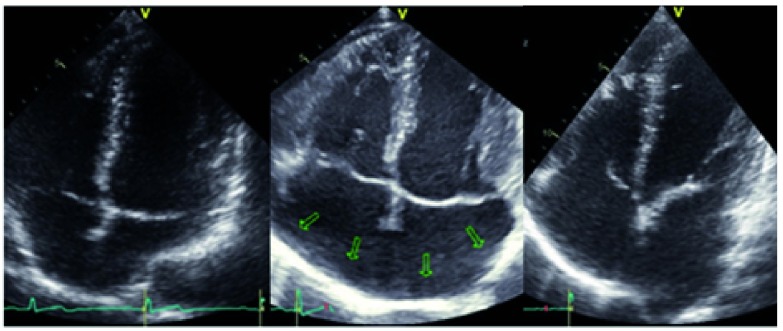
Pathological cardiac remodelling (especially of right and left atrium) of a 48 years old triathlete with EIAH. In 2011, the participant showed a normal size of the right and left atrium. An atrial enlaragement occured 2014 (arrows) after a period of high intensity training (echocardiography in 4 chamber view during atrial fibrillation). Even after cardioversion (2014) into sinus rhythm (7 days in sinus rhythm) he retains larger atrial cavities as in 2011.
